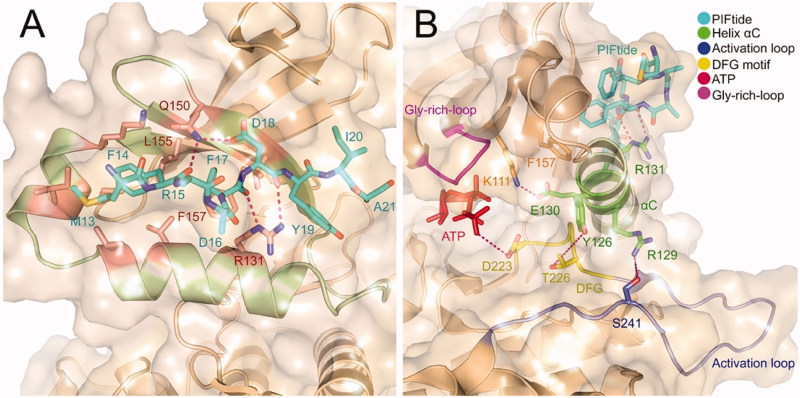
(A) Ribbon representation of the X-ray structure of PDK1-PIFtide complex (PDB ID: 4RRV). The residues that form the PIF-pocket are shown in salmon, while the other residues from PIFtide are shown in cyan. (B) Ribbon representation of the connection of the helix αC with the activation loop, DFG, and Gly-rich-loop. The helix αC and related residues are shown in green, DFG and related residues are shown in yellow, activation loop and related residues are shown in slate, and Gly-rich-loop is shown in magenta.
